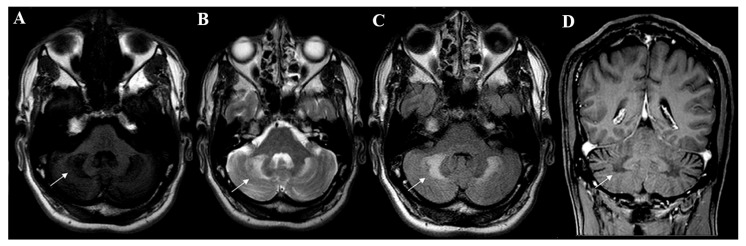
Neuroimaging patterns observed in CTX. Axial brain MRI disclosed signal change in deep cerebellar white matter and dentate nucleus (white arrows) which were hypointense on T1W (A) and hyperintense in T2W (B) and FLAIR sequences (C). Coronal brain MRI showed hypointensity in the deep cerebellar white matter on T1W imaging (D).
